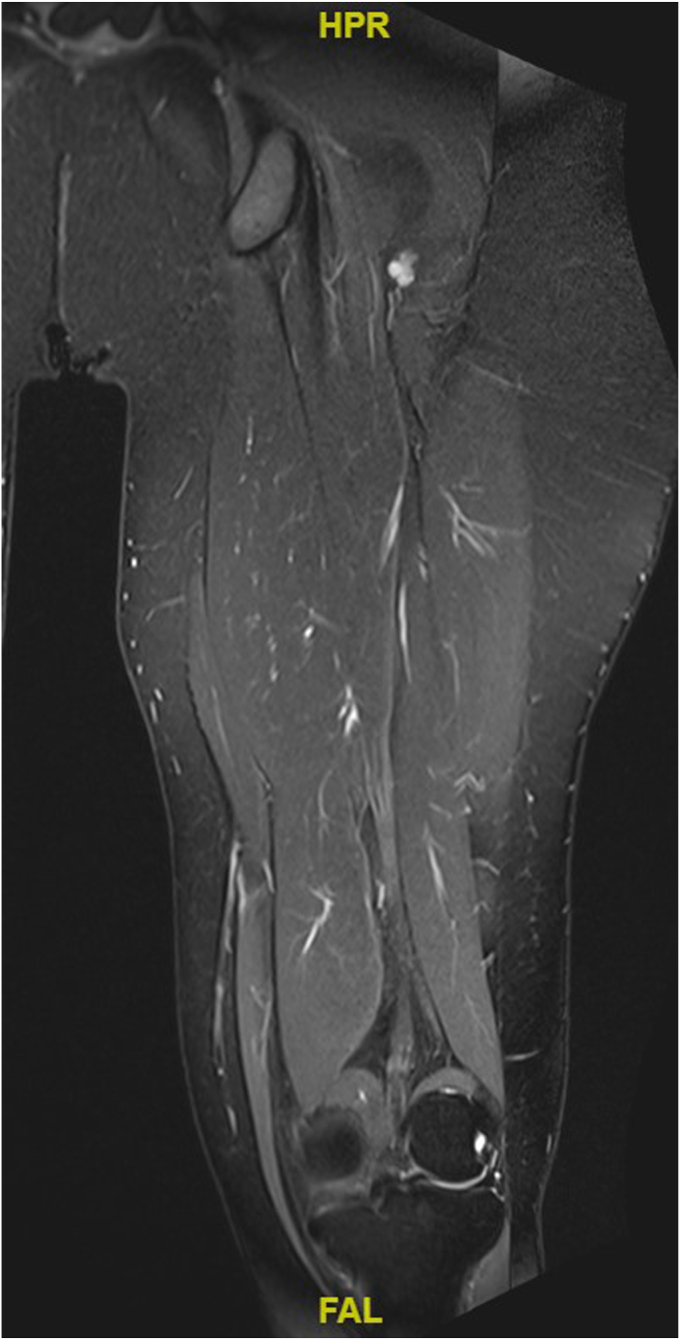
T2 MRI of the *left* thigh, showing a hyperintense lesion posterior to the proximal femur most consistent with a peripheral nerve sheath tumor. *MRI* = magnetic resonance imaging.
